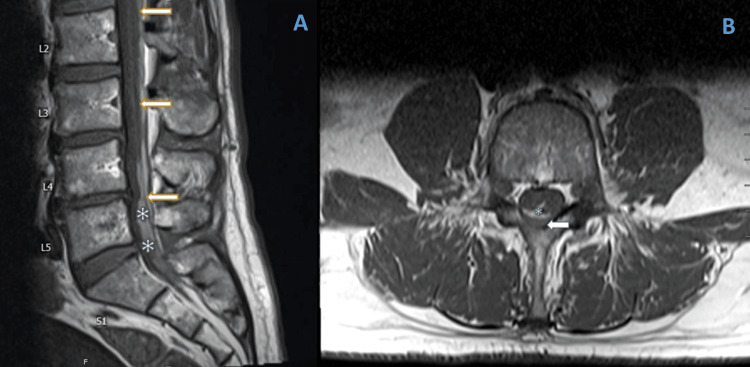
The T1-weighted sagittal (Panel A) and axial (Panel B) MR image of the spine demonstrates a subdural collection of blood (white arrows) extending from T12-L1 to sacral level and subarachnoid collection of blood (asterisks) extending from L3-4 to the termination of the thecal sac at S2.
